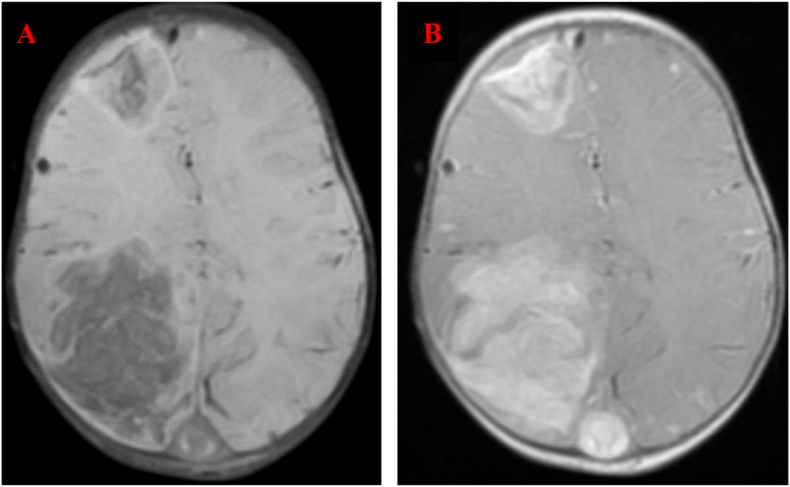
A) magnetic resonance imaging (MRI) T1-sequence showed right temporoparietal hypersignal B) MRI Fat-Saturation sequence showed a fading of the hypersignal.
